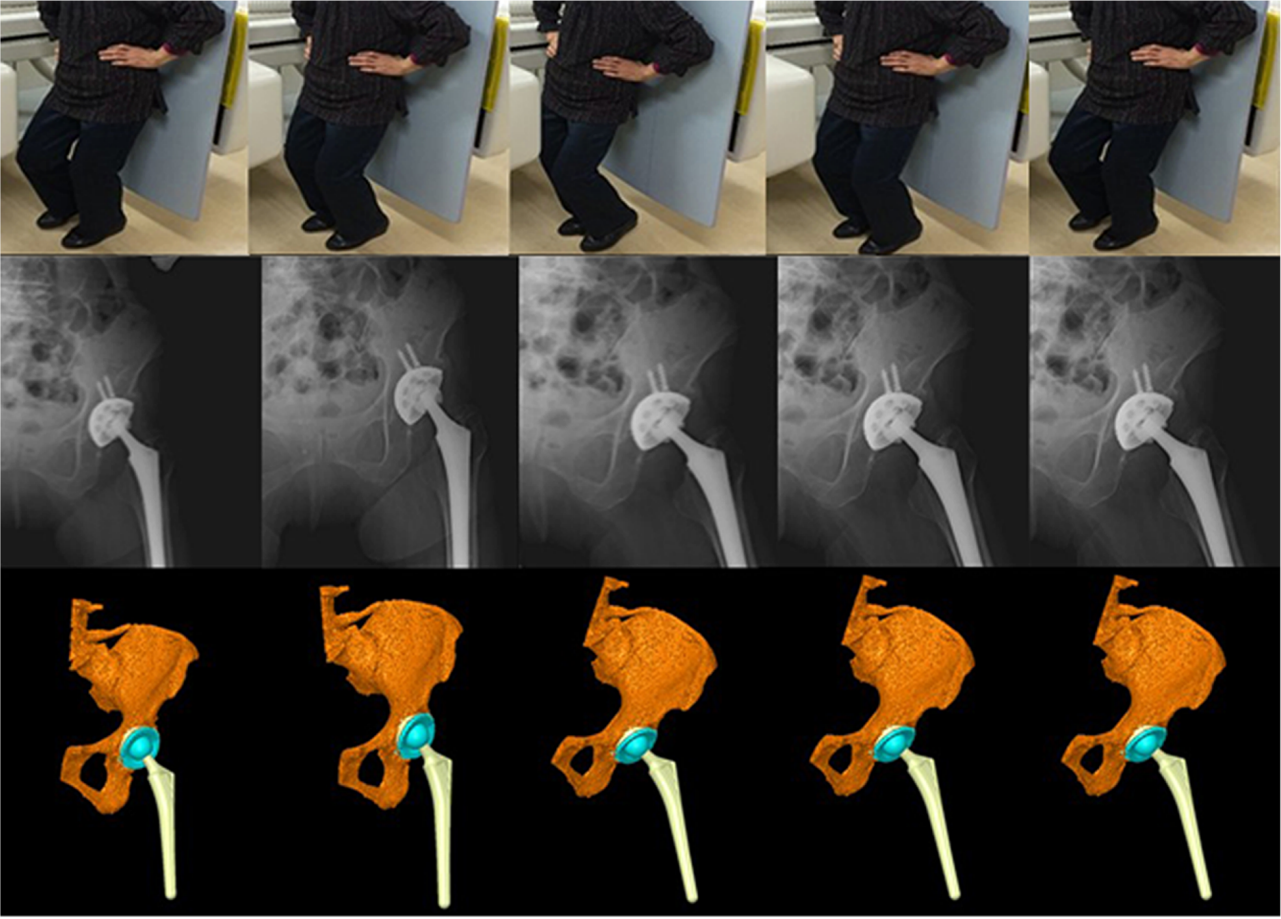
The hip motions during the hula dance movement kao were captured as continuous X-ray images using a flat panel X-ray detector (*middle stand*) to reconstruct three-dimensional images of the replaced hip joint (*lower stand*) using image-matching techniques
